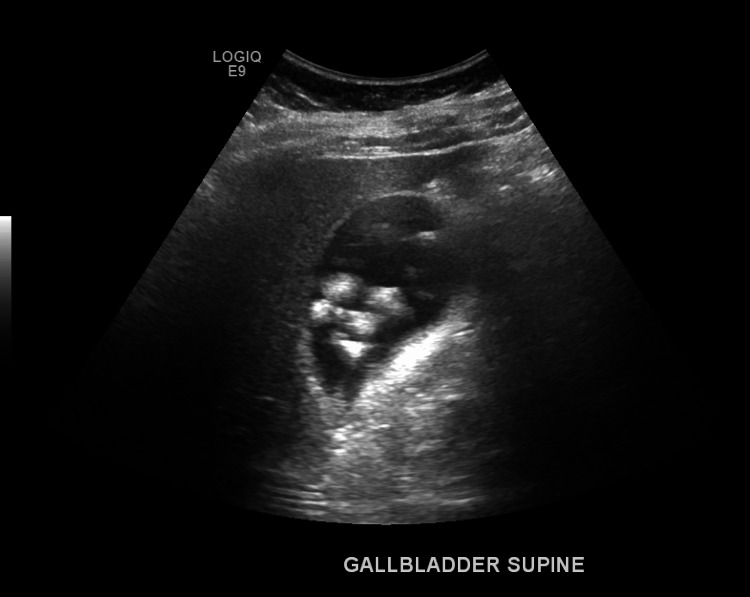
RUQ abdominal ultrasound revealed a non-distended GB with multiple shadowing gallstones. The GB wall measured 3 mm with no thickening, no wall hyperemia, and no pericholecystic fluid. RUQ, right upper quadrant; GB, gallbladder
